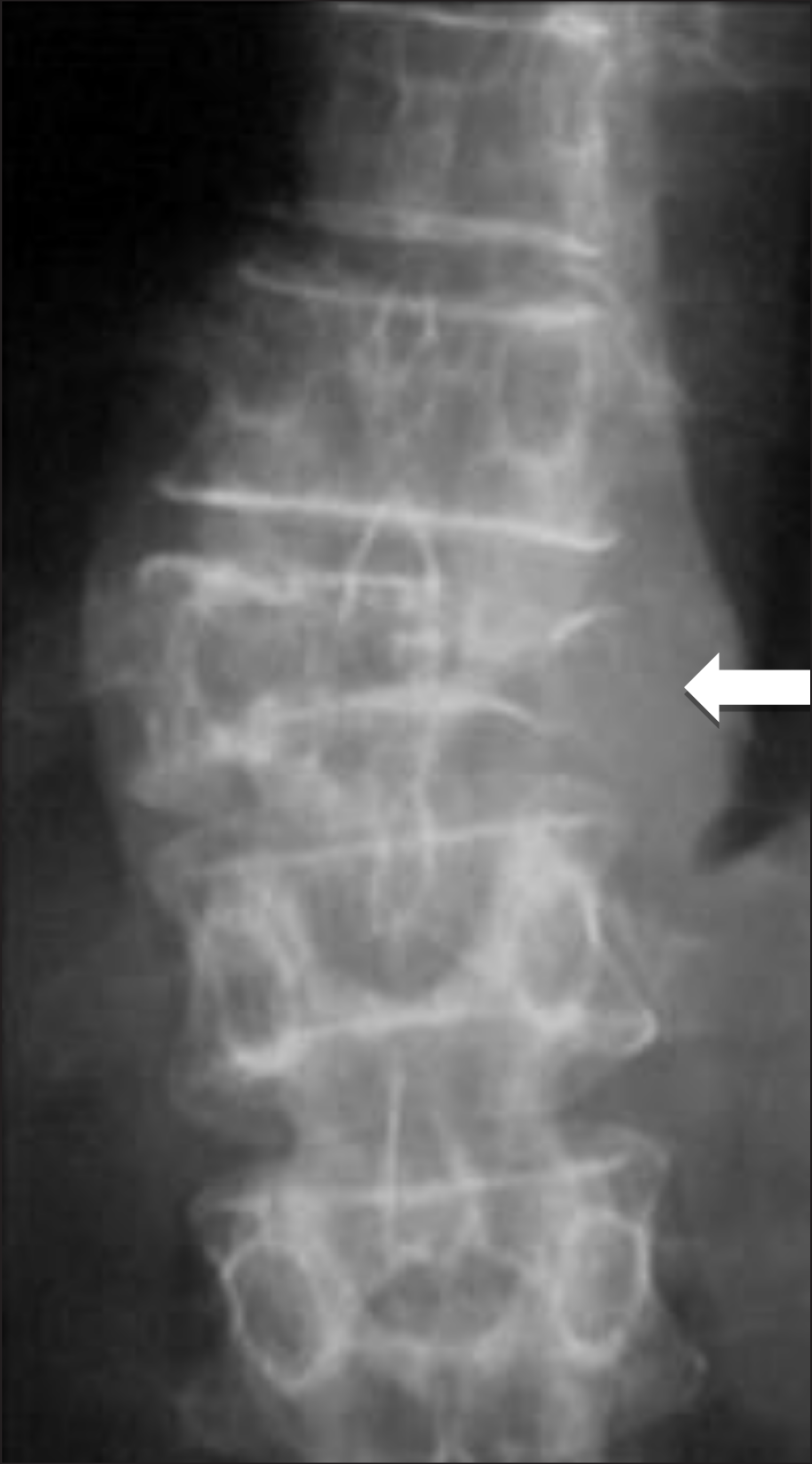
Multiple myeloma. Plain radiograph of spine shows an osteolytic lesion with cortical breakthrough, vertebral collapse and soft tissue mass (arrow).
